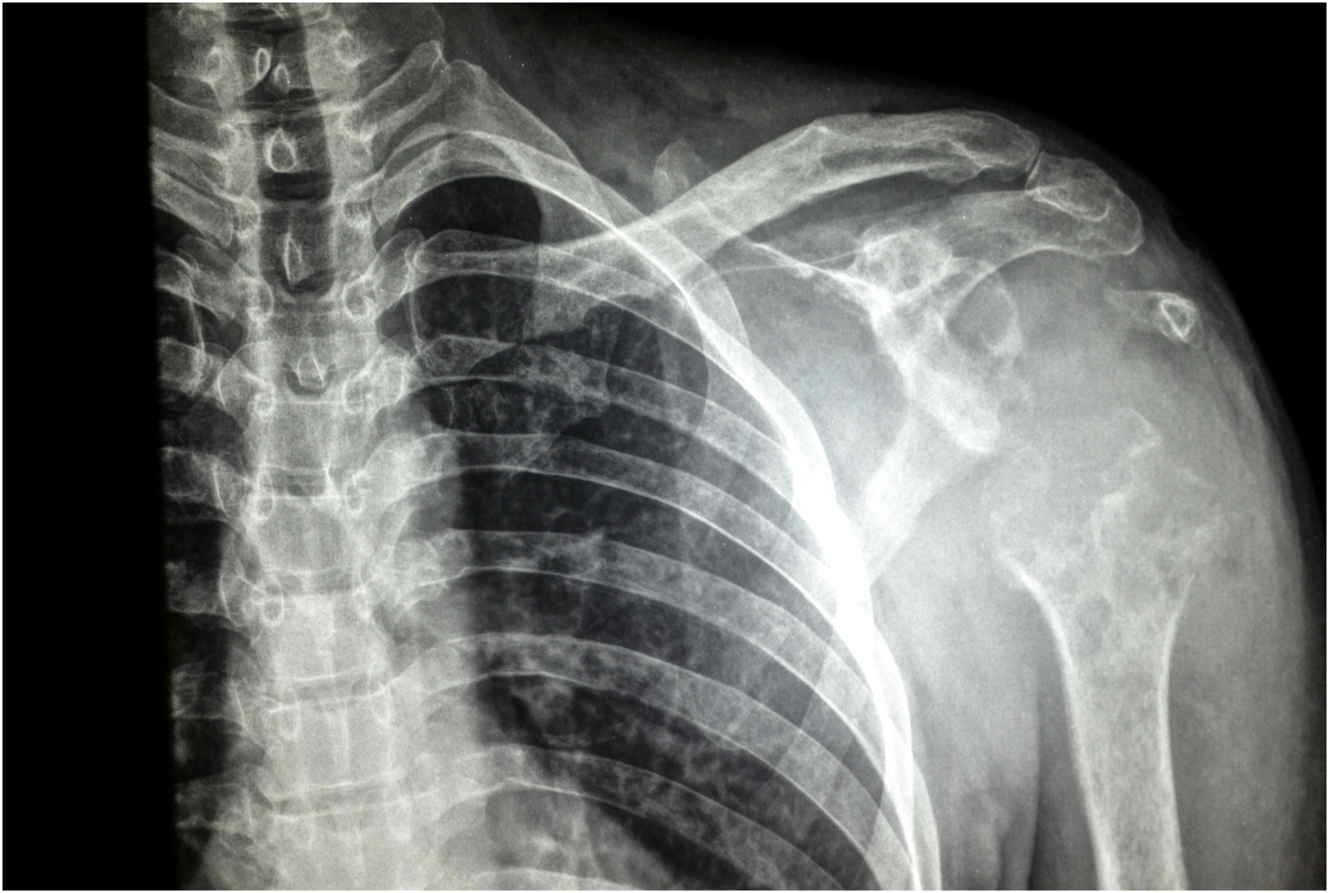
X-ray of the left shoulder joint showing soft tissue mass, complete destruction of the humeral head, and multiple cavities in glenoid cavity and coracoid process.
